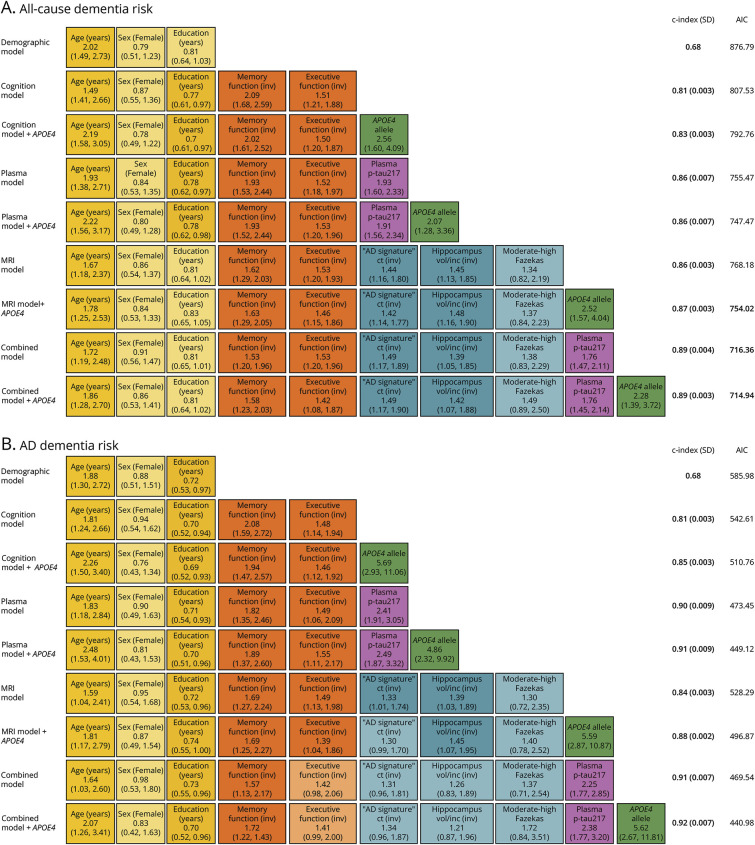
Comparative Performance of Multivariable Models for Assessing the Risk of All-Cause Dementia (A) and AD Dementia (B) in Individuals With SCD Models included different combinations of variables: demographics (age, sex, education), cognitive measures (memory and attention/executive functions), plasma biomarker (p-tau217), *APOE4* allele status, and MRI-based imaging markers (cortical thickness in “AD-signature” region, hippocampal volume adjusted by icv, and Fazekas score for white matter hyperintensities). Cognitive domain scores, plasma p-tau217 levels, and MRI measures were standardized into *z*-scores using linear regression models adjusted for relevant covariates (age for all measures, and education additionally for cognitive tests), based on a reference population of cognitively unimpaired individuals from BioFINDER-1 and BioFINDER-2 cohorts. To ensure interpretability, *z*-scores for cognitive and MRI variables were inverted, so that higher values consistently indicated worse performance or greater atrophy, aligning with the direction of plasma p-tau217. All models were fitted in the same sample of individuals with SCD from BioFINDER-1 and BioFINDER-2 cohorts (n = 469), after multiple imputation of missing data. Continuous variables were scaled before imputation. For each variable in each model, HR with 95% CI is presented. HRs with nonsignificant *p* values are shown using a lighter color in the figure. Model performance is indicated by the Harrell concordance index and SD (C-index [SD]), with the SD reflecting variability across imputations. SD is not reported for the demographic model because all variables included in this model were complete and needed no imputation. AIC is reported as a measure of model fit. Lower AIC values and higher C-index indicate better model performance. AIC = Akaike information criterion; AD = Alzheimer disease; ct “AD-signature” = cortical thickness of the “AD signature” area (this area includes the measurements for entorhinal, inferior-temporal, middle temporal, and fusiform regions); HR = hazard ratio; icv = intracranial volume; (inv) = inverted; p-tau = phosphorylated tau; vol = volume; SCD = subjective cognitive decline.
